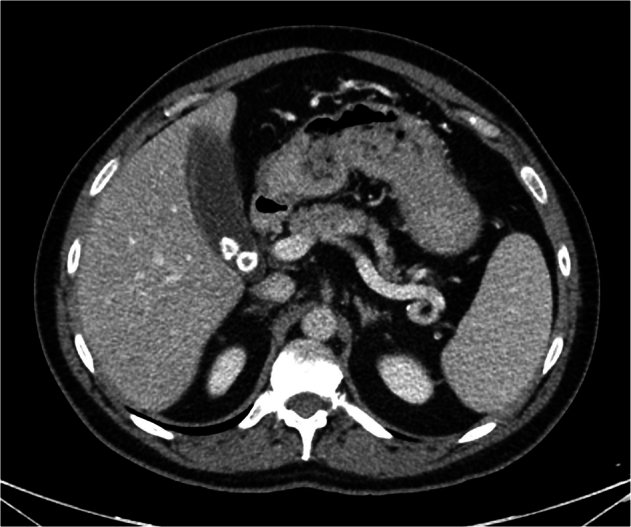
CT scan of the abdomen showing cholelithiasis. GPT-4V correctly identifies the image modality (“CT scan”) and the anatomical location (“Abdomen”). The pathology identified by GPT-4V is “Splenic laceration with subcapsular hematoma and hemoperitoneum,” meaning it missed the correct pathology of cholelithiasis and hallucinated the splenic pathology
